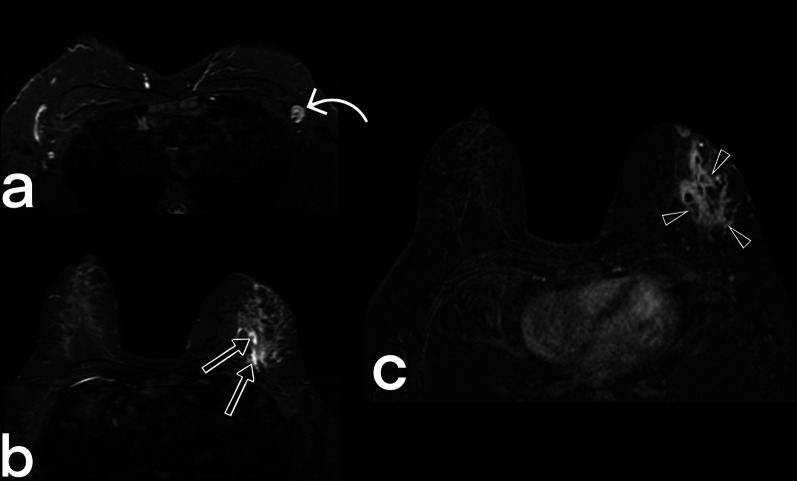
MR images of a 32-year-old female. Axial T2-weighted fat-suppressed (a, b) and T1-weighted fat-suppressed contrast-enhanced images (c) reveal a signal increase and heterogeneous non-mass enhancement with regional type distribution in the left breast (arrowheads), and abnormal axillary lymph nodes (curved arrow). Small fluid collections are also noted (arrows). BIRADS 4
